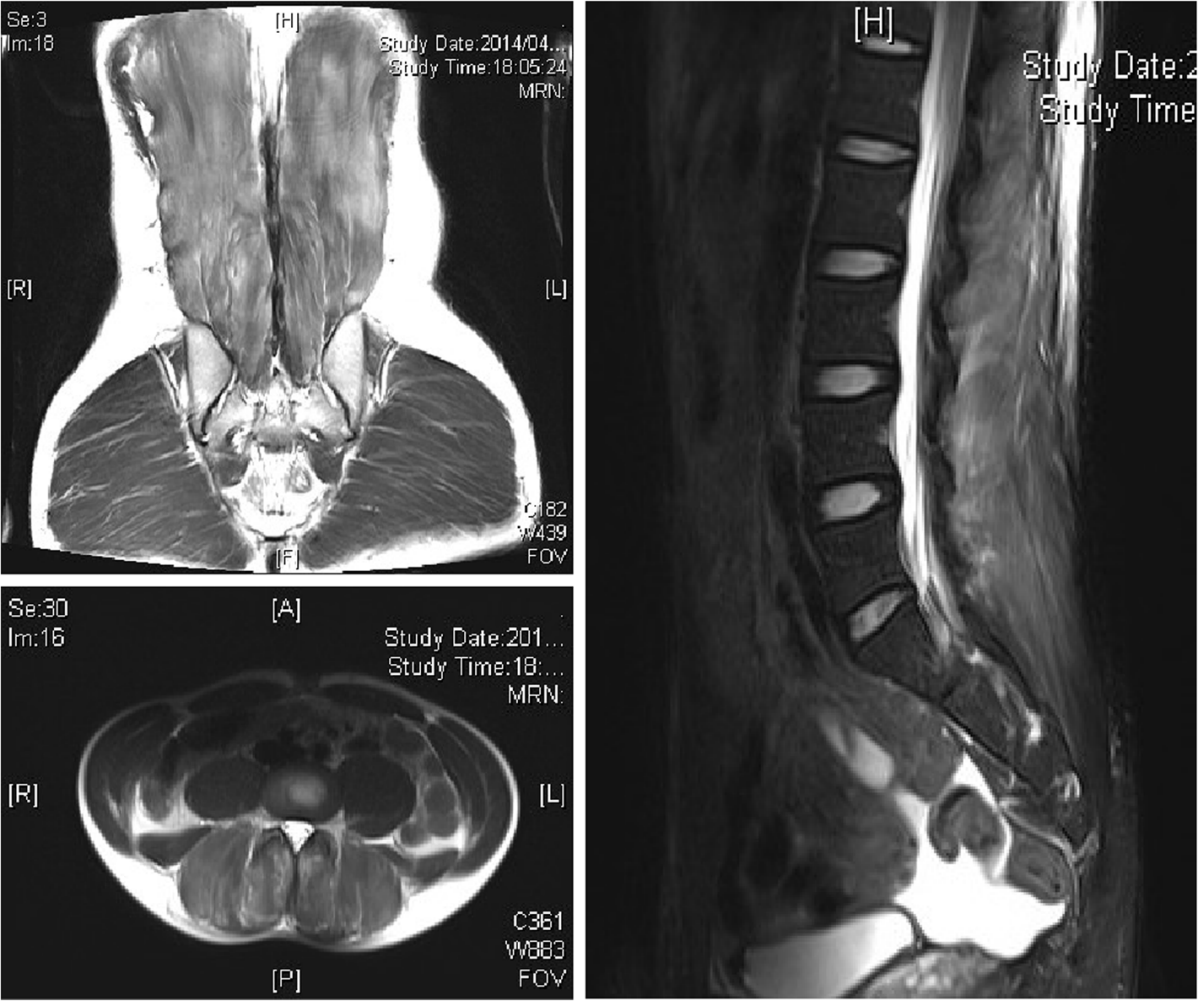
Magnetic resonance imaging at admission. The paravertebral muscles were edematous
